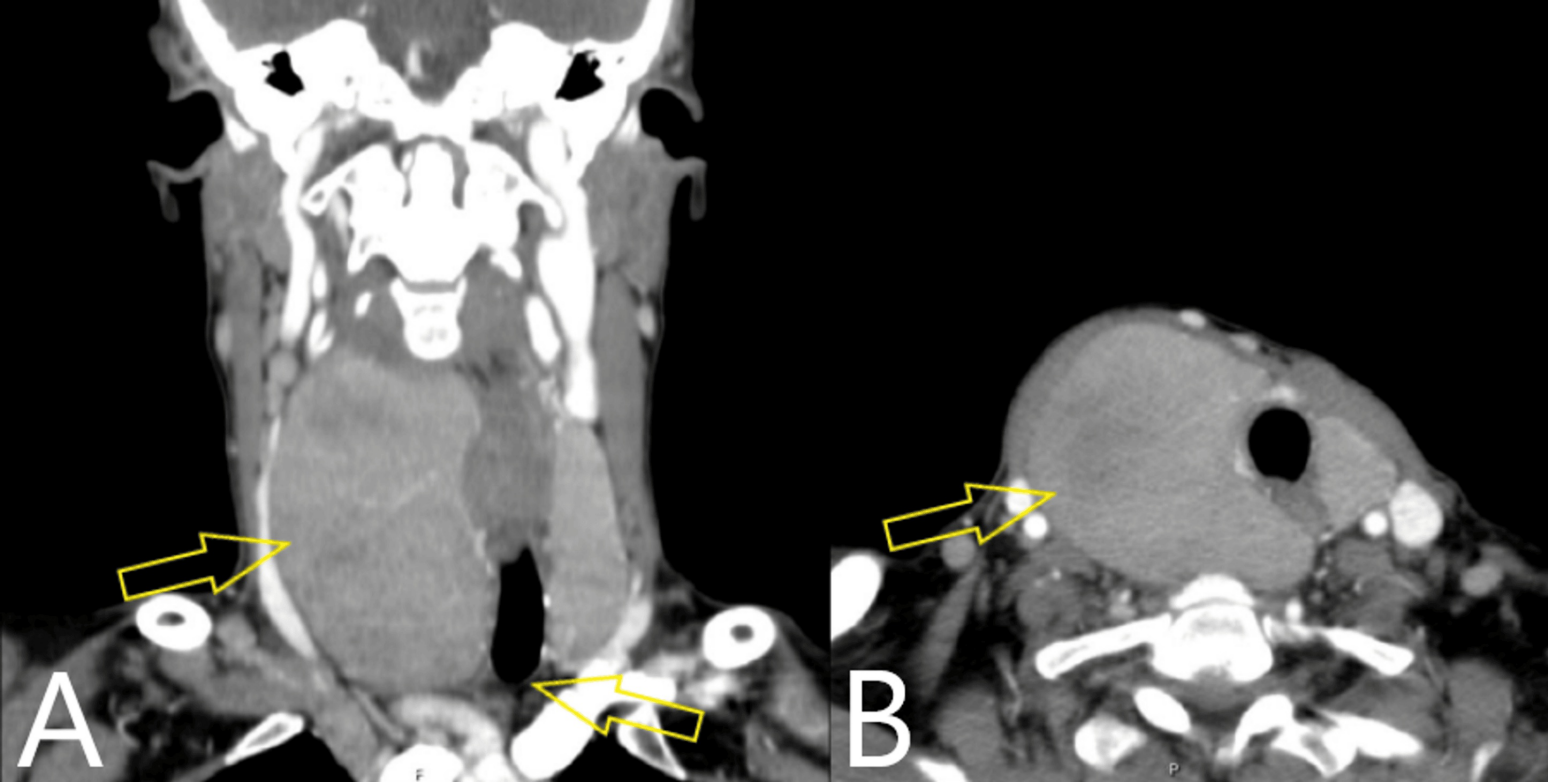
Coronal (A) and axial (B) CT images of the neck and chest showing an enlarged right thyroid lobe extending into the superior mediastinum with marked compression and deviation of adjacent structures including the trachea, esophagus, and cervical vessels (yellow arrows).
